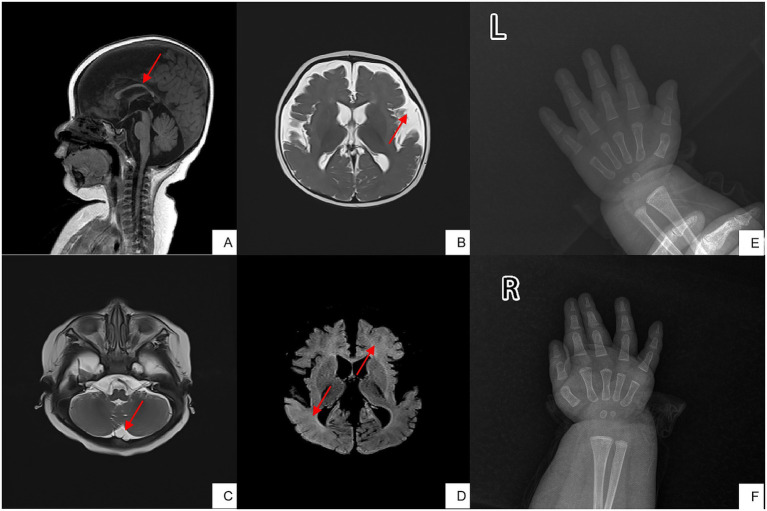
Imaging findings of the patient with Ververi–Brady syndrome caused by *QRICH1* gene variant. (A–D) Brain magnetic resonance imaging (MRI). (A) Sagittal T1WI showing thin corpus callosum; (B) Axial T2WI showing widened frontotemporal extra-axial spaces and dilated lateral ventricles; (C) Axial T2WI showing enlarged cisterna magna; (D) Axial FLAIR showing diffusely increased signal intensity in bilateral cerebral white matter. (E,F) X-rays of both hands showing no obvious bony abnormalities.
